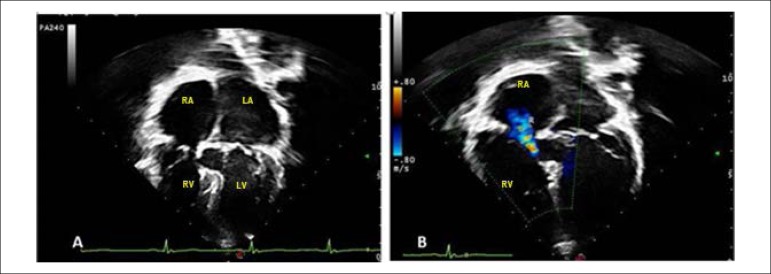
Echocardiogram shows a 4-chamber view of a subcostal section with normal-sized cardiac cavities, with closed defects and good atrioventricular valve coaptation, in A and B. RA: right atrium; LA: left atrium; RV: right ventricle; LV: left ventricle.
